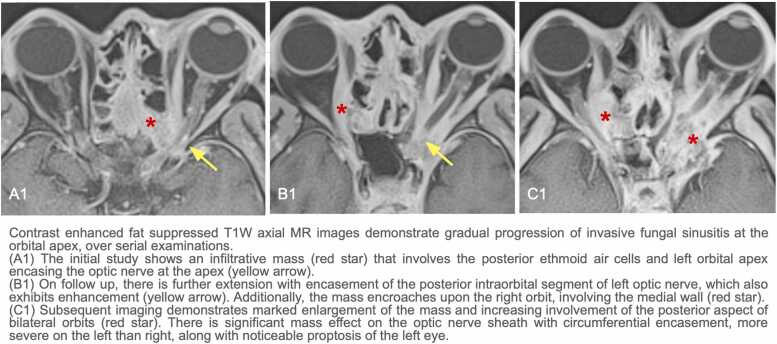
Contrast enhanced fat suppressed T1W axial MR images demonstrate gradual progression of invasive fungal sinusitis at the orbital apex, over serial examinations.
